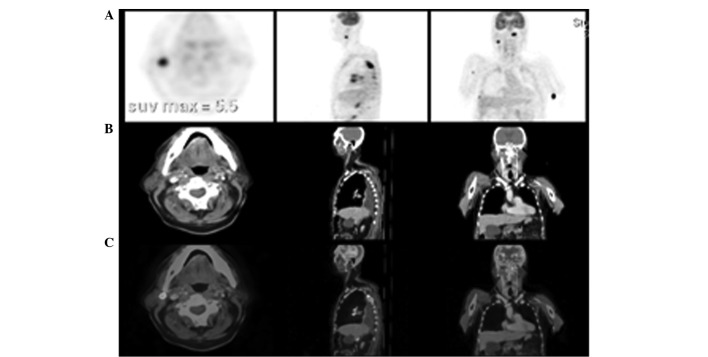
(A) ^18^F-fludeoxyglucose-positron emission tomography (^18^FDG-PET) and (B and C) fused PET-computed tomography (CT) revealing areas of hypermetabolism consistent with diffuse metastatic dissemination to the spine, right parotid gland, lungs and adrenal glands, as well as hilar, mediastinal and peritoneal lymph nodes. SUV, standardized uptake value.
